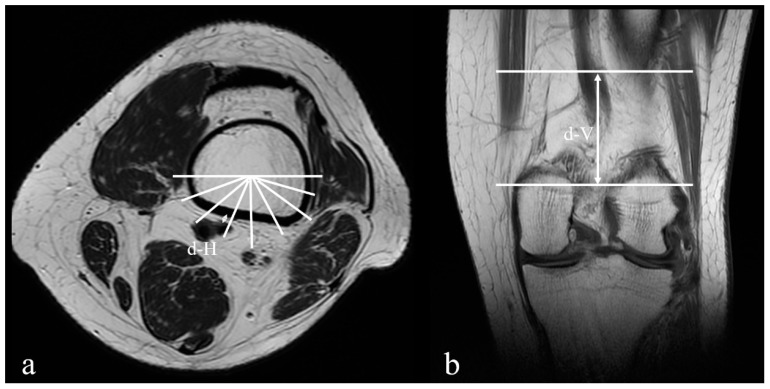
The example of the measurements in magnetic resonance images of a 28-year-old male: (a) the closest distance (d-H) between the popliteal vessels and the femoral cortex is 1.22 mm, and the position of the popliteal vessels adjacent to the femoral cortex is section C; (b) the distance (d-V) between the AT and the axial level of “d-H” is 66.98 mm.
